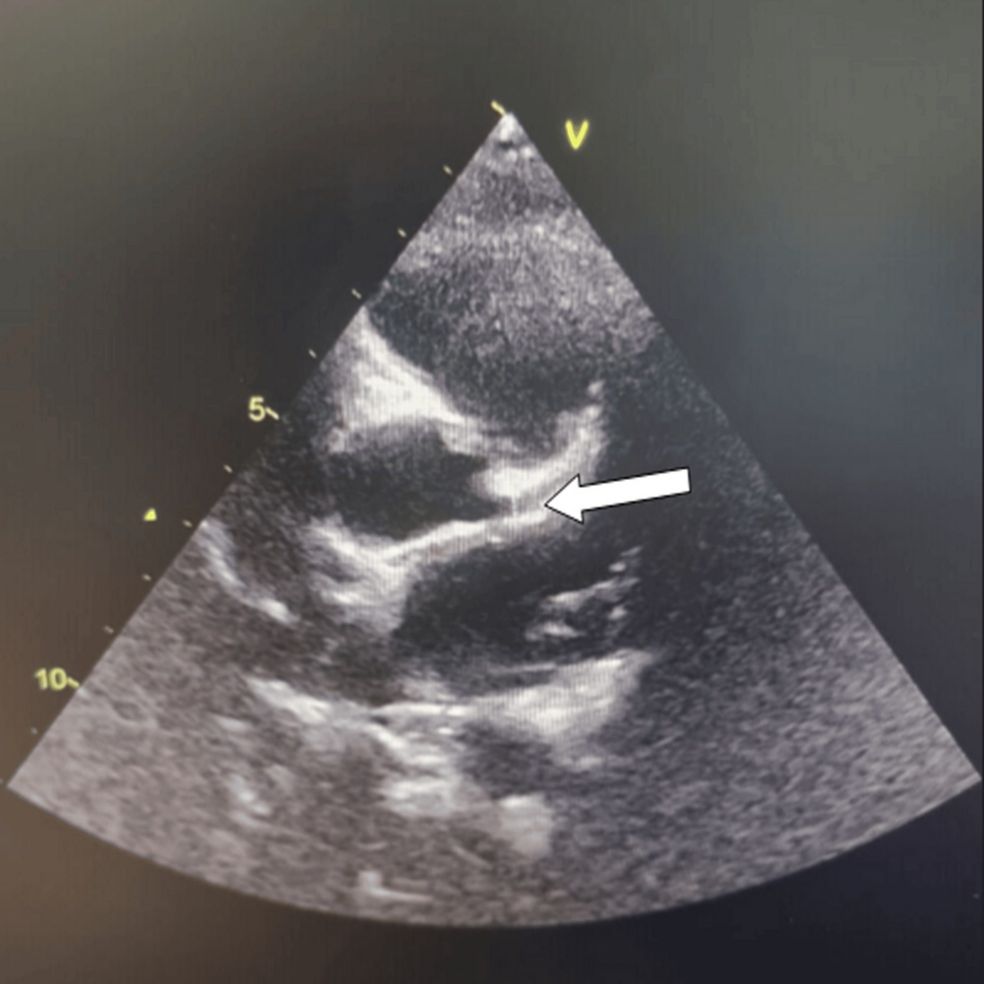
Echocardiography showed a dilated left coronary artery at 4mm.
